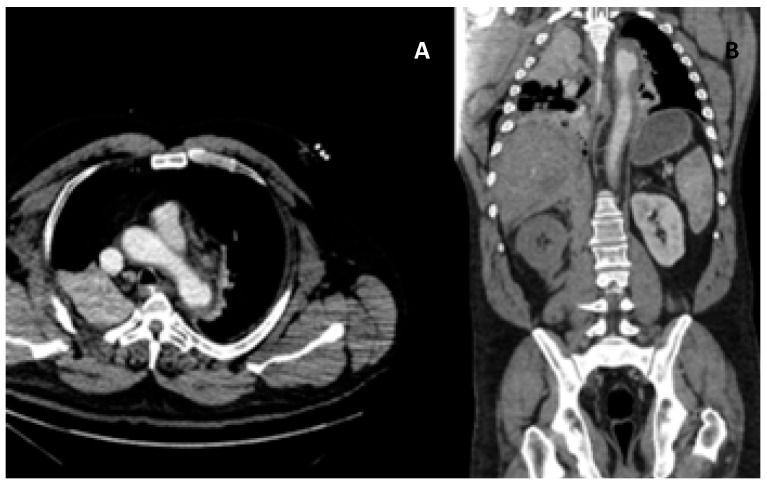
Collision trauma. Axial computed tomography (CT) (A) and coronal reconstruction (B) identify focal dissection and periaortic hematoma in the isthmus region.
